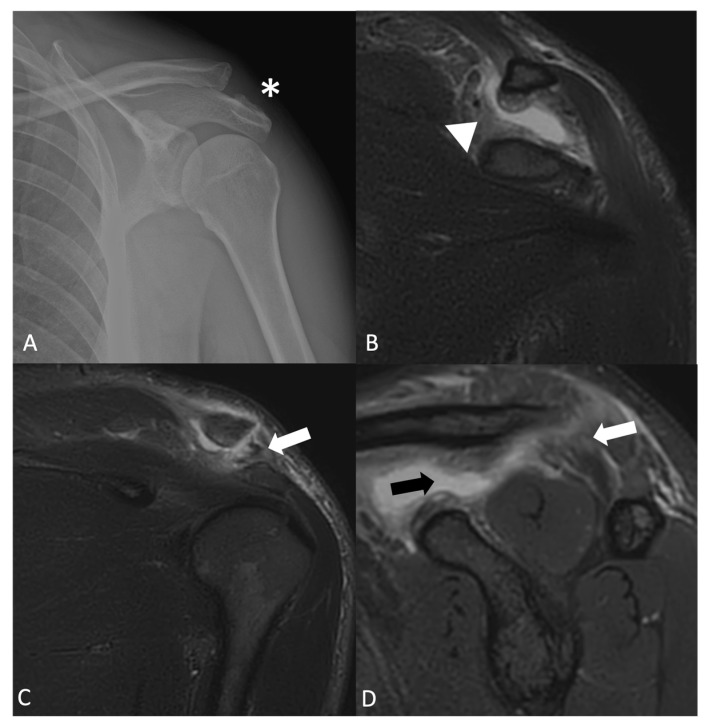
(A) AP X-ray, (B,C) coronal STIR, and (D) sagittal STIR in a patient with type III acromioclavicular dislocation according to the Rockwood classification. There is a rupture of both the acromioclavicular (white arrows) and coracoclavicular ligaments, trapezoid ligament rupture (black arrow), and conoid ligament rupture (arrowhead), causing complete dislocation of the joint (asterisk).
